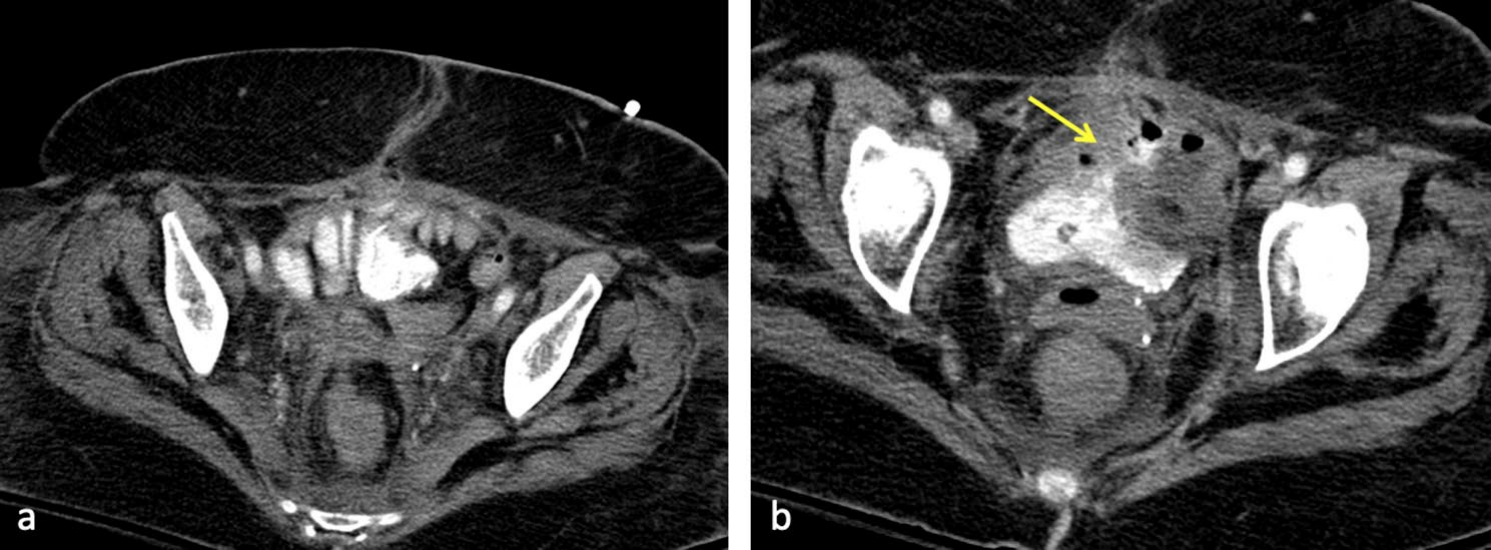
66-year-old female with rectal cancer status post chemoradiation presented with small bowel obstruction. CT of the abdomen and pelvis with oral contrast a demonstrates small bowel superior and ventral to the bladder with surrounding inflammatory changes. An air-filled tract extends from small bowel loops to the bladder (b, yellow arrow). Air and dense contrast in the bladder is enteric in origin
